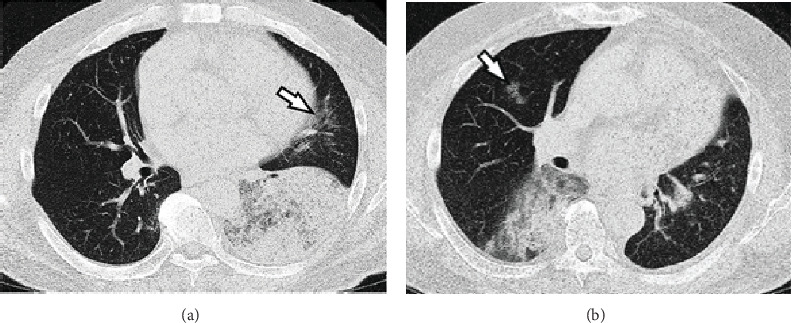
Axial CT images. (a) A chest CT image obtained 7 days after the initial visit shows a large infiltrative shadow, as well as a small infiltrative shadow that is suspected to be a skip lesion (arrow). (b) A chest CT image obtained 2 months after left lobectomy shows an infiltrative shadow with a skip lesion (arrow) in the right lung.
